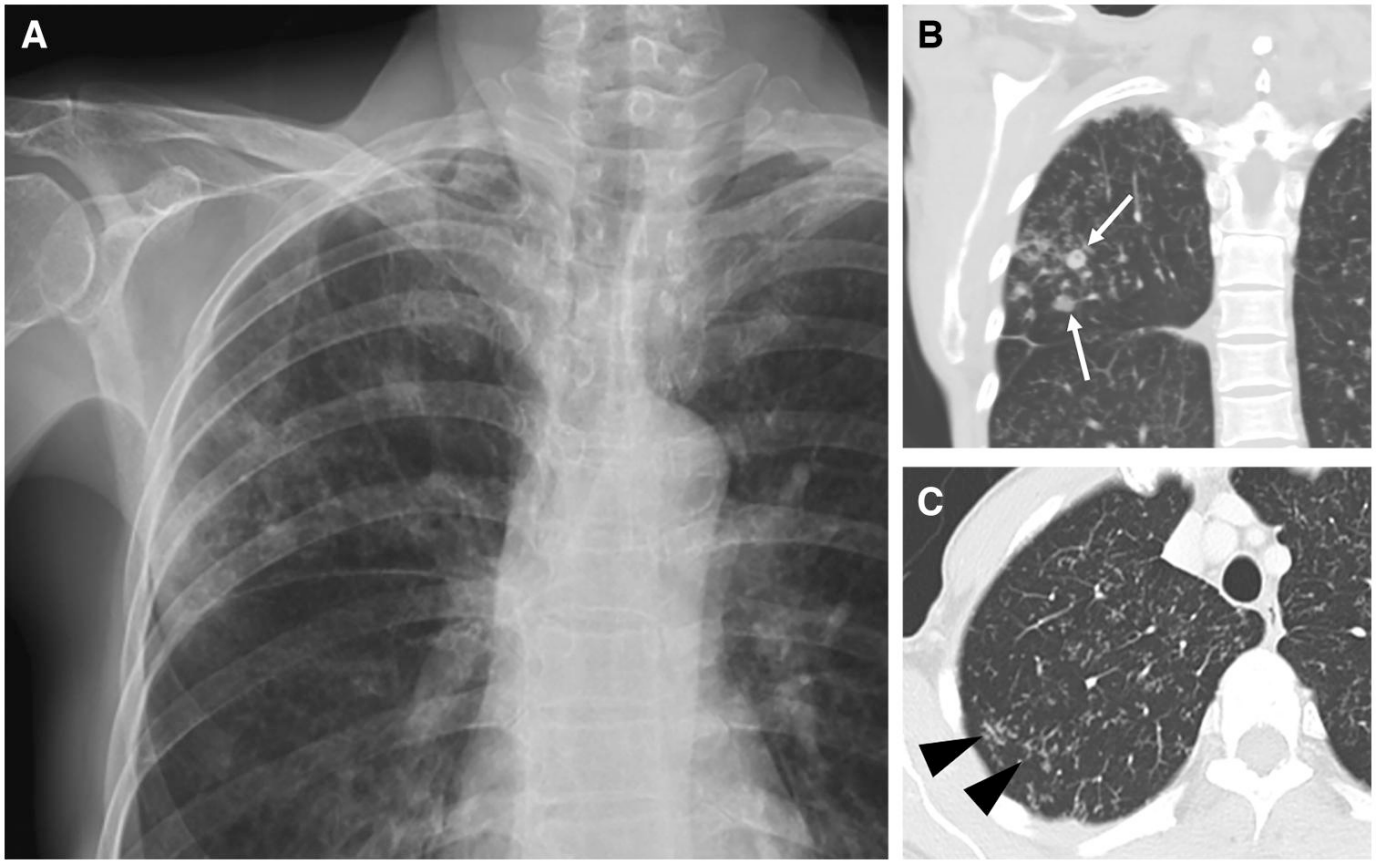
Pulmonary tuberculous nodules in a 61-year-old woman. (A) Frontal chest radiograph and (B) coronal CT image show reticulonodular infiltration in the right upper lobe, with several nodules (white arrows). (C) Axial CT image shows tree-in-bud opacities in central zone as well as in the peripheral posterior aspect of the lung (black arrowheads).
